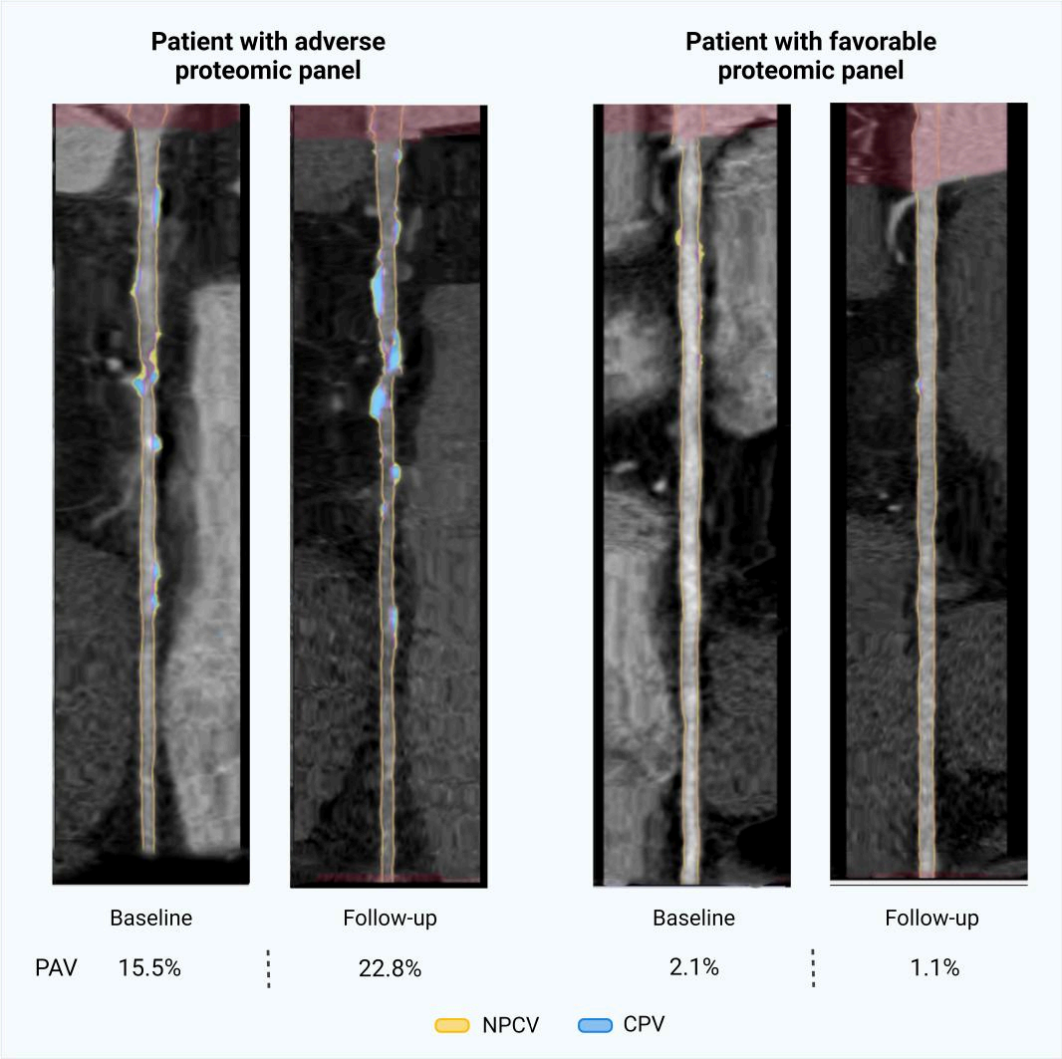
Visual comparison of PAV plaque progression. Example of AI-QCT analysis of the RCA in a patient with adverse (left) and favourable (right) inflammatory proteomic panels. PAV, percent atheroma volume; NCPV, percent non-calcified percent volume; CPV, percent calcified volume; AI-QCT, artificial intelligence–guided quantitative coronary computed tomography angiography analysis; RCA, right coronary artery. Created with BioRender.com.
